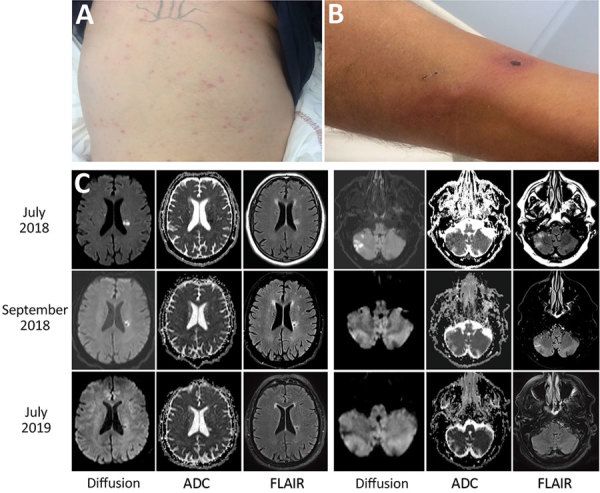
Clinical manifestations and cerebral magnetic resonance imaging of a 66-year-old man with *Rickettsia sibirica mongolitimonae*–associated encephalitis, southern France, 2018. A) Maculopapular rash. B) Black eschar and rope-like lymphangitis on the right leg. C) Magnetic resonance imaging with diffusion (B1000), ADC, and FLAIR. In July 2018, cytotoxic lesions were observed intra-axially and in the white matter of right cerebellar hemispheres with FLAIR hypersignal and with low ADC signal. In September 2018, these cytotoxic lesions regressed in diffusion with the appearance of a necrotic cavity facing the roof of the left lateral ventricle. In July 2019, disappearance of diffusion anomalies. Small necrotic cavity with after-effects on FLAIR and ADC signals. ADC, apparent diffusion coefficient; FLAIR, fluid-attenuated inversion recovery.
